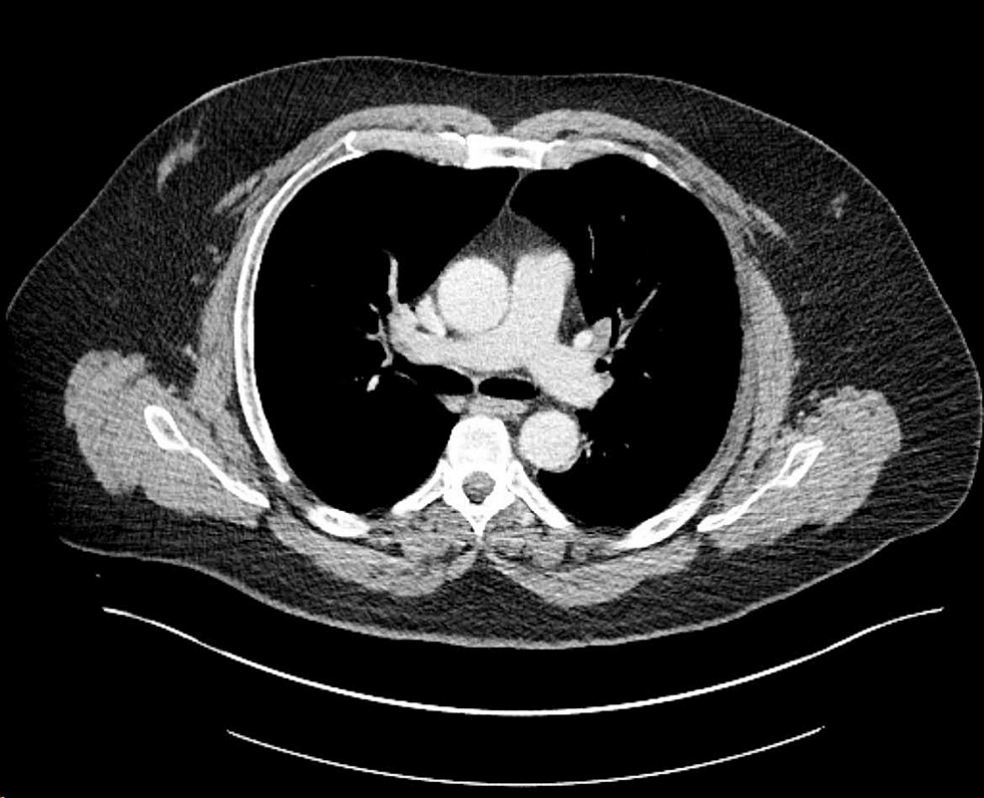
CT of the chest showing bilateral hilar lymphadenopathy
